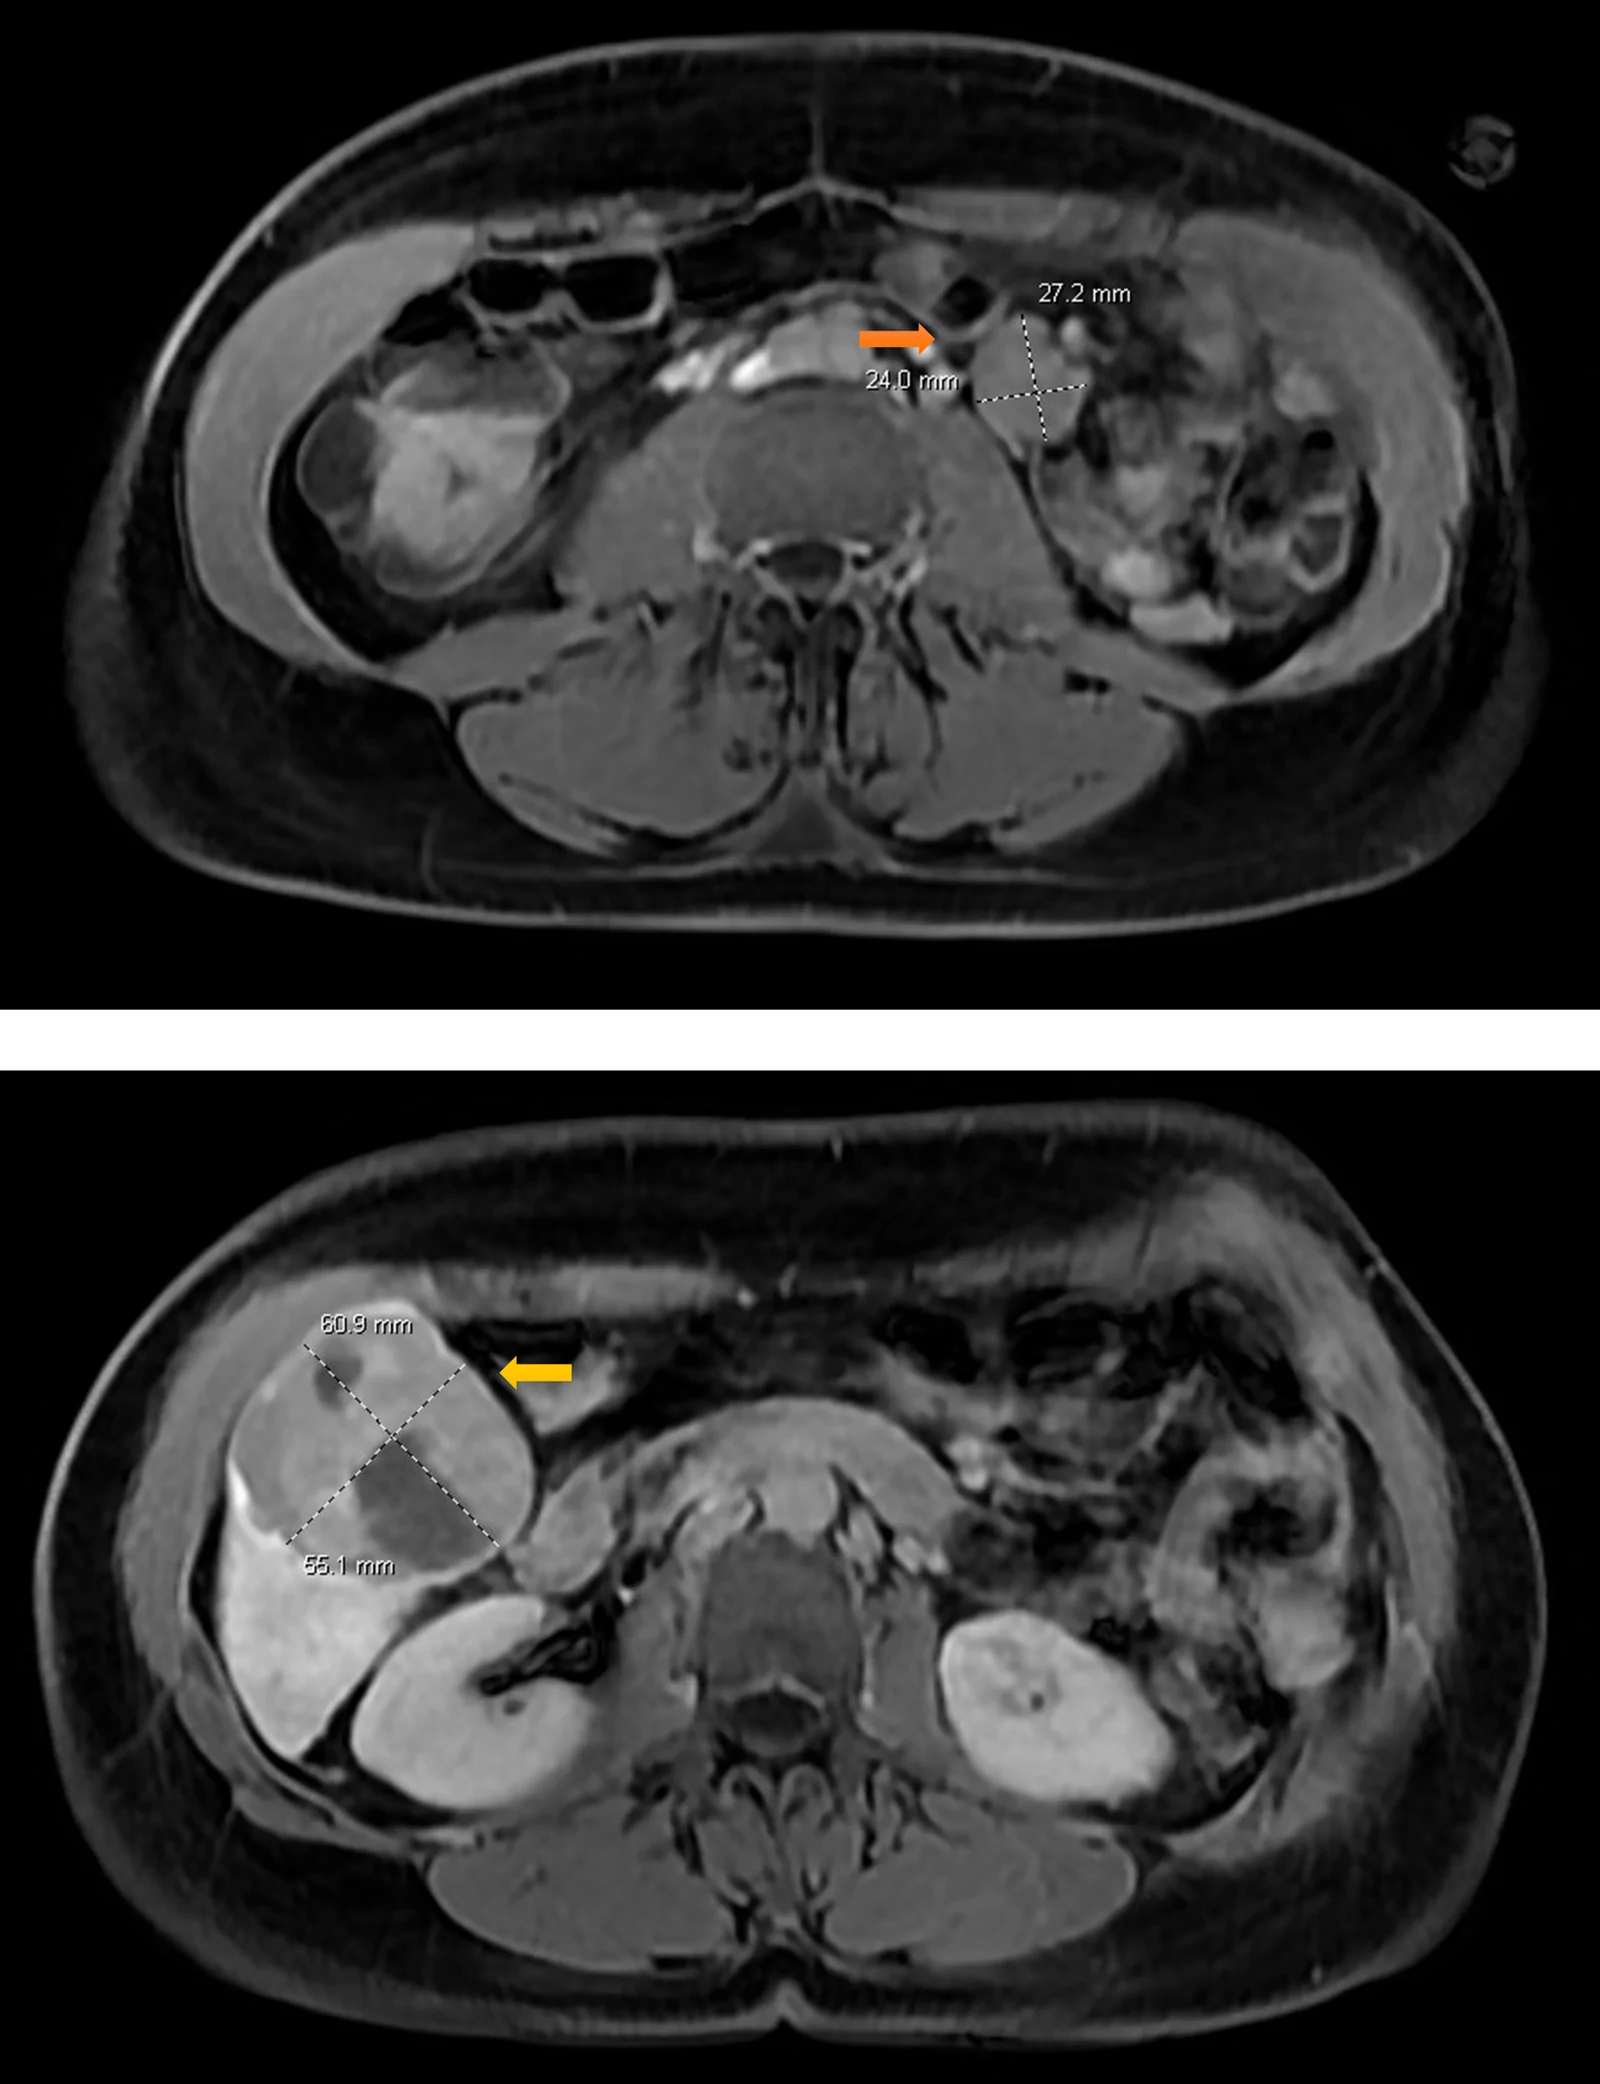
Findings on Magnetic Resonance Imaging of Abdomen Magnetic resonance imaging of the liver showing an enhancing mesenteric mass in the left hemi abdomen measuring approximately 2.7 × 2.4 cm (red arrow) and a heterogeneously enhancing mass (yellow arrow) arising from the hepatic segment 5 extending into the adjacent mesentery abutting the ventral abdominal wall measuring approximately 5.5 × 6.1 cm. The hepatic mass demonstrates focal areas of variable intrinsic T1 and T2 hyperintensity, suggesting cystic and hemorrhagic/proteinaceous contents.
